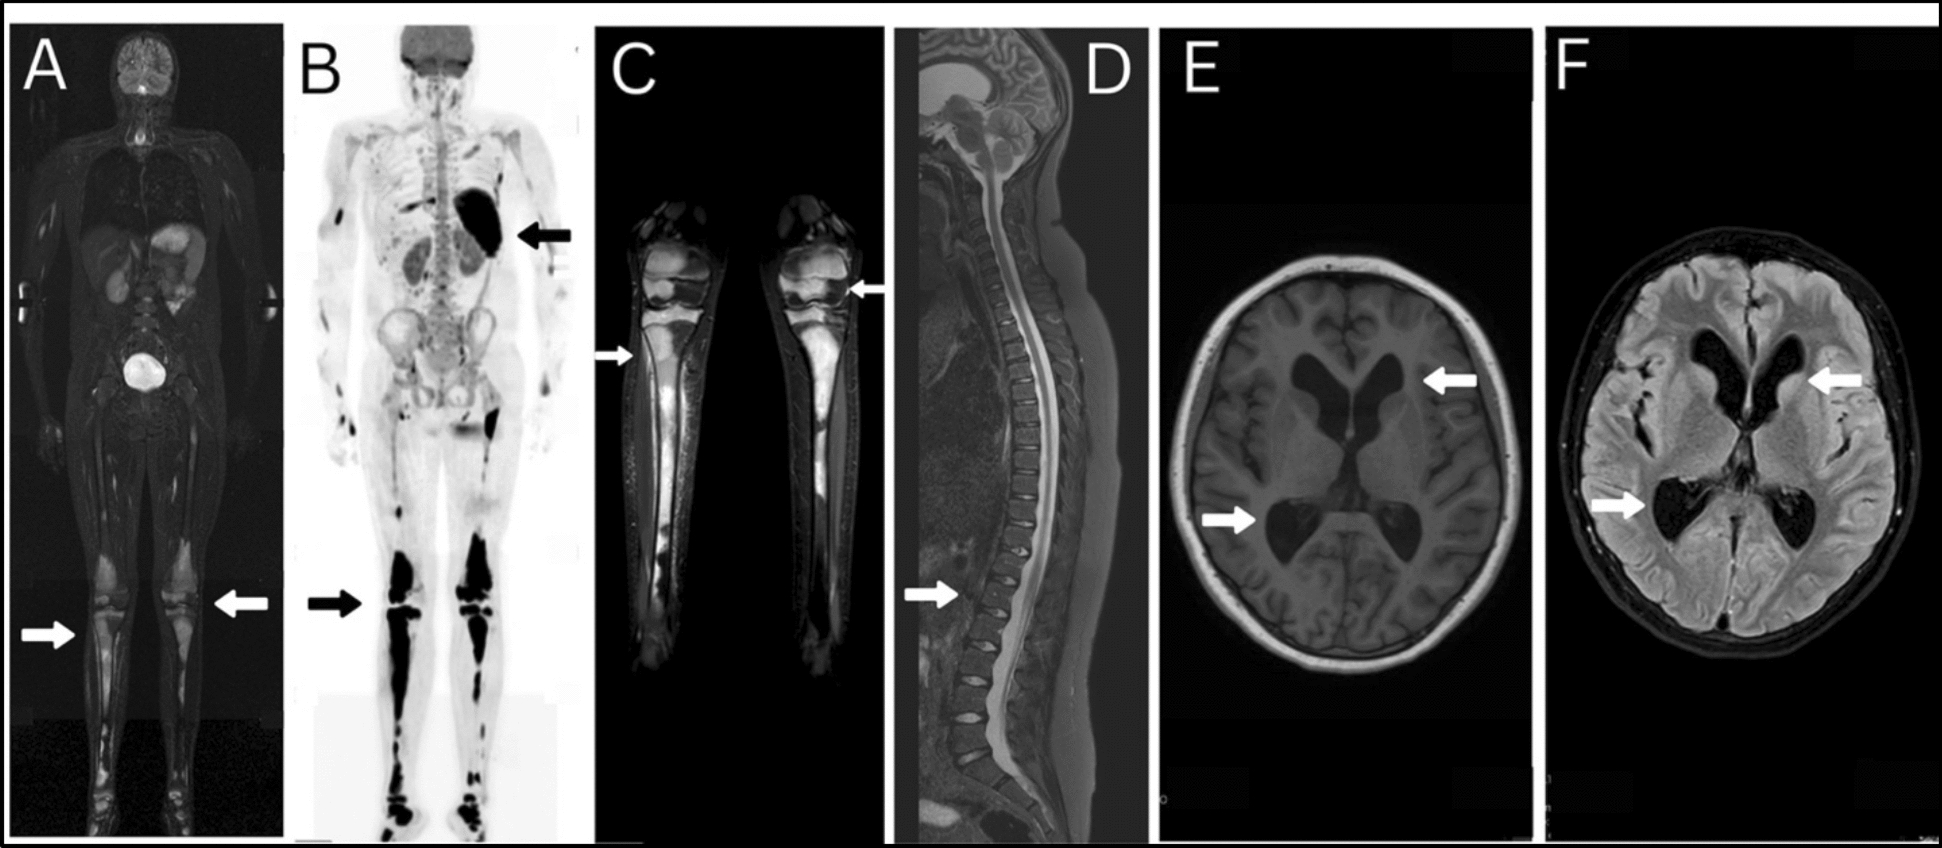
Magnetic resonance imaging, positron emission tomography, and central nervous system imaging in rhabdomyosarcoma with relapsed non-Hodgkin lymphoma. A (age 11 years) Whole-body coronal short tau inversion recovery magnetic resonance imaging showing diffuse bone marrow infiltration, particularly in the proximal and distal tibias (white arrows), consistent with metastatic involvement. B (age 11 years) Whole-body positron emission tomography scan reveals hypermetabolic activity (black arrows) in the right tibia, femur, and pelvis, confirming the presence of multiple skeletal metastases. C (age 11 years) Bilateral lower leg coronal magnetic resonance imaging T1 images, highlighting low signal intensity lesions in both tibias (white arrows), suggestive of tumor infiltration and correlating with positron emission tomography findings. D (age 11 years) Sagittal T2-weighted spinal magnetic resonance imaging demonstrates abnormal signal intensity and swelling in the thoracic spinal cord (white arrow), raising suspicion of leptomeningeal spread or spinal involvement. E (age 14 years) Axial T1-weighted magnetic resonance imaging shows ventriculomegaly and sulcal efacement, suggesting obstructive hydrocephalus due to central nervous system involvement of non-Hodgkin lymphoma. F (age 14 years) Axial fluid-attenuated inversion recovery magnetic resonance imaging reveals periventricular and basal ganglia hyperintensities, likely related to leukemic infiltration or treatment-induced leukoencephalopathy. White arrows in E and F showing where sarcoma and lymphoma were observed
